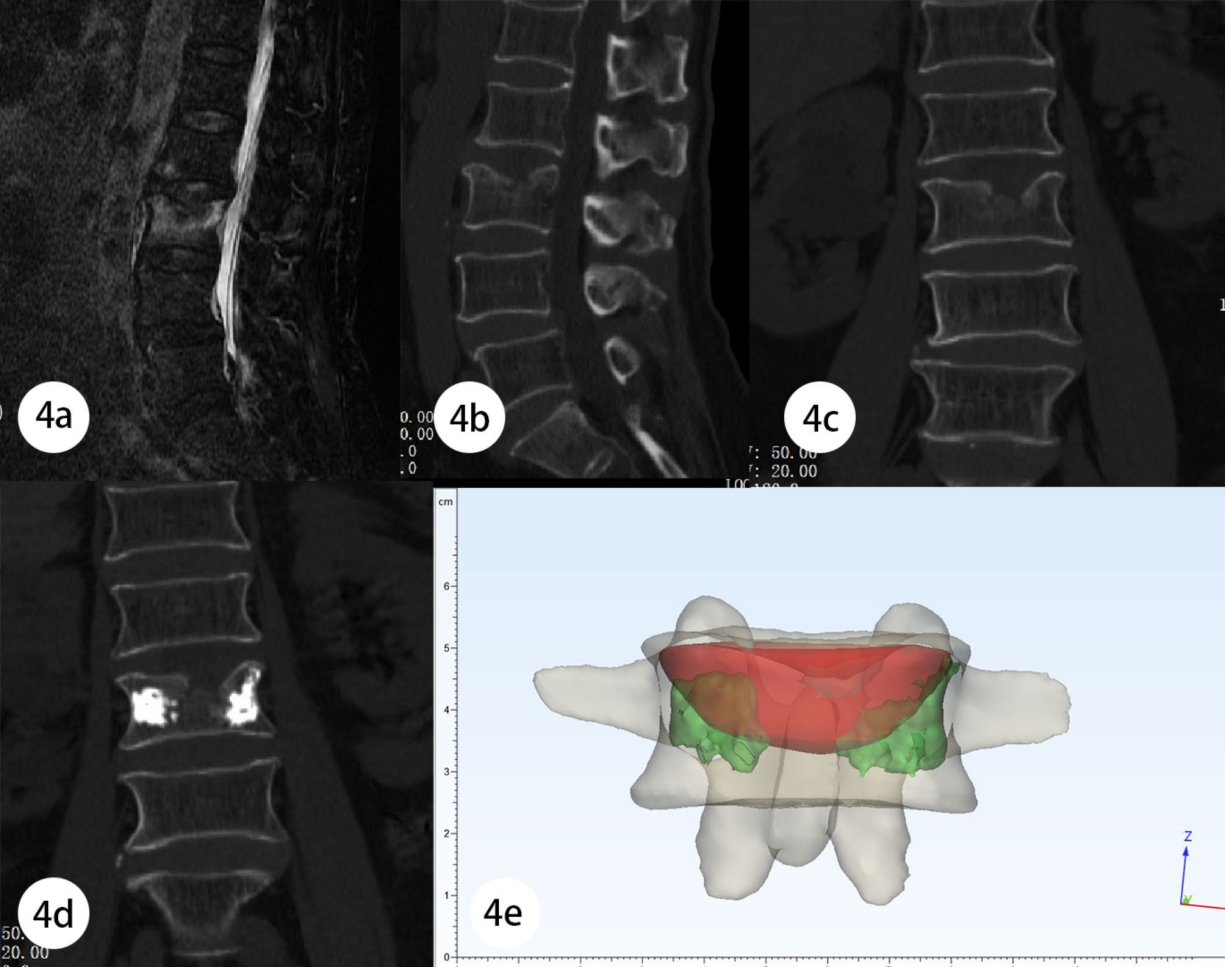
Case1: 4a The preoperative fat suppression image of lumbar spine MRI showed SNs with Modic I changes in the cephalic endplate of L3 vertebra. 4b、c SNs were displayed on sagittal and coronal images of preoperative CT. 4d Postoperative CT showed poor distribution of bone cement. 4e Reverse reconstruction model, calculated R_f_=13.85%
